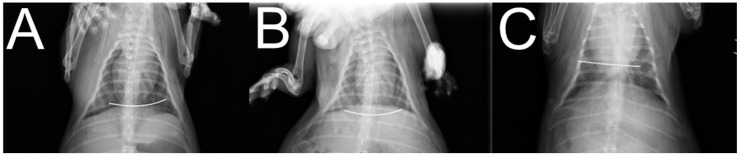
X-ray images of implanted PLA Nuss bars, (A) 1 month, (B) 2 months, (C) 3 months post-implantation (a metal wire was inserted at the core of the bars for easy identification).
